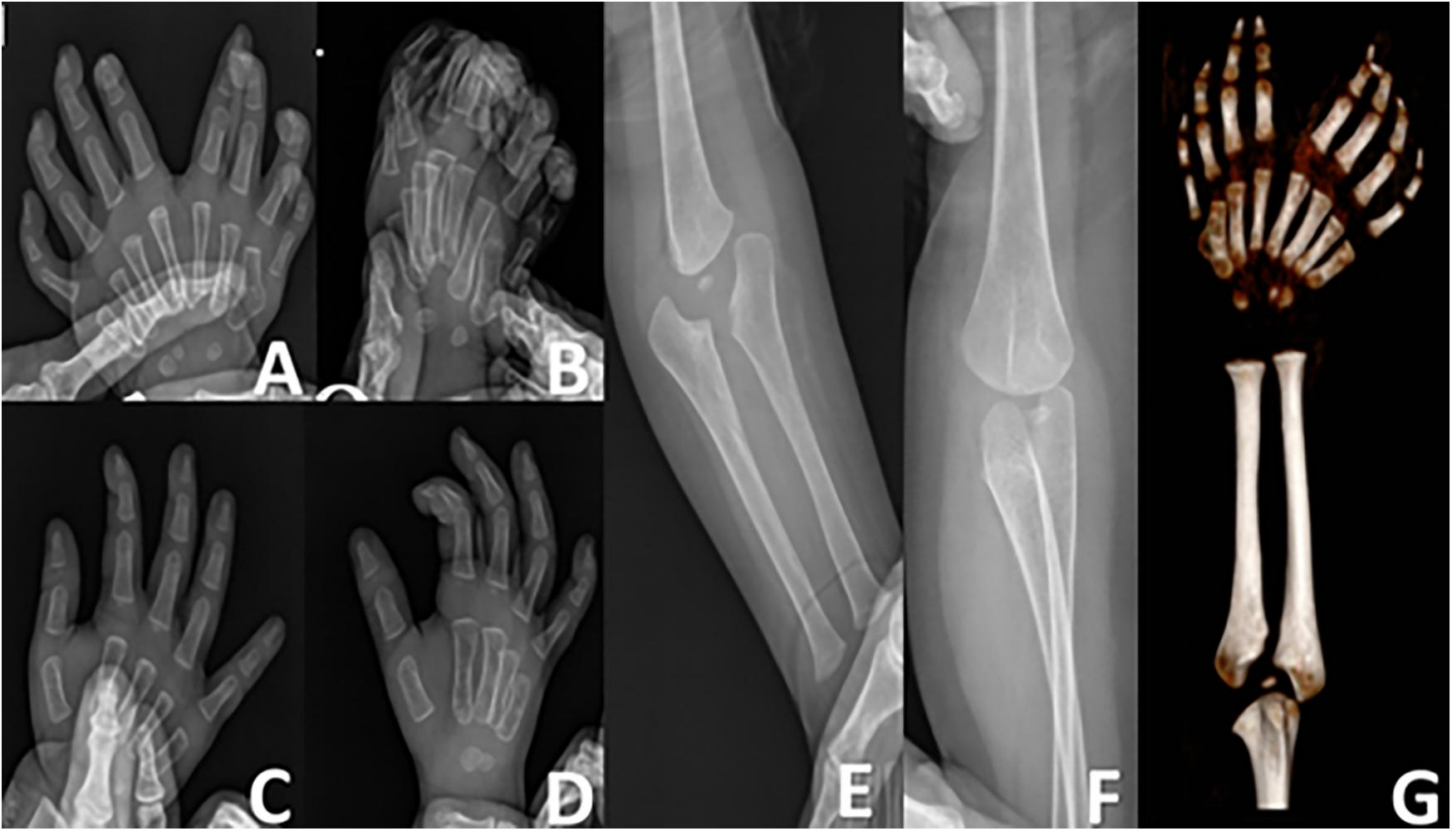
(A,B) orthogonal and oblique radiographs of the left hand. (C,D) Orthogonal and oblique radiographs of the right hand. (E,F) Radiographs of the left elbow joint. (G) Three-dimensional computed tomography reconstruction of the left forearm and hand.
